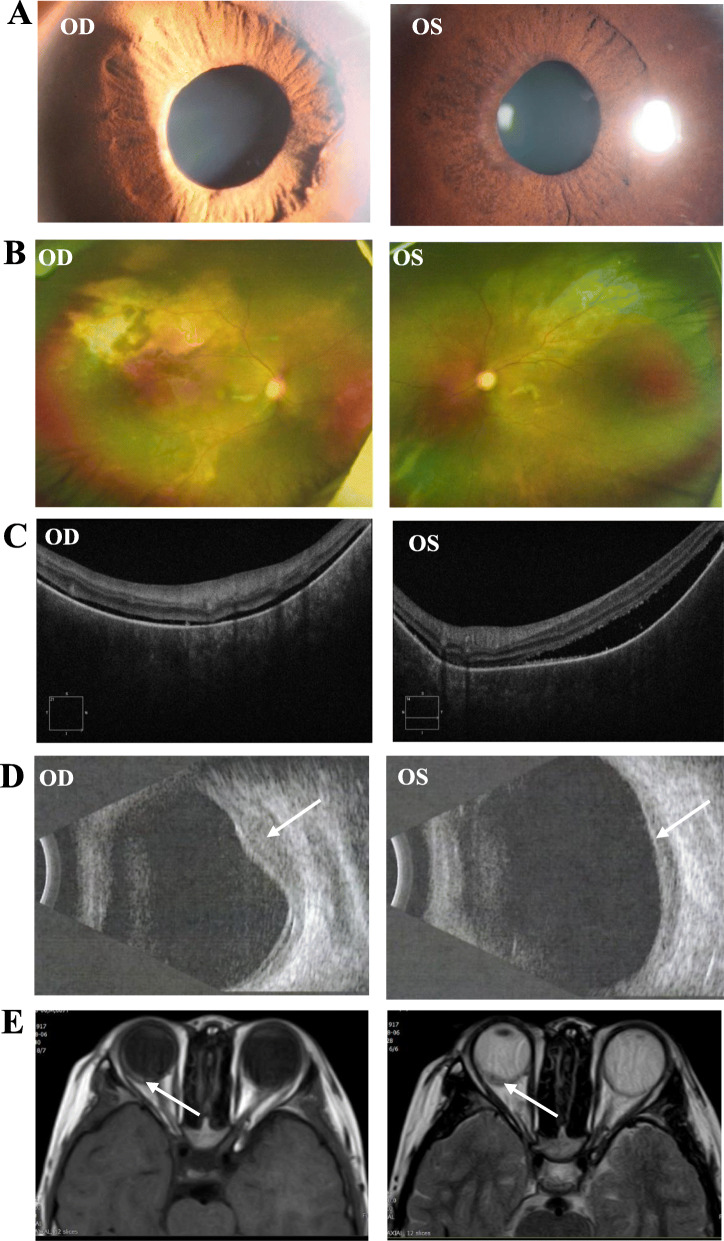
Images of comprehensive ophthalmic examinations. a The bilateral pupils were irregular and unable to dilate. b Funduscopy showed vasculitis and exudation bilaterally. c Bilateral retinal detachment was observed in optical coherence tomography. Choroidal vasculature was dramatically absent. d A dome-shaped mass bulging from choroid to retina was observed in B scan. e Diffuse thickening of bilateral choroids was verified with magnetic resonance imaging
